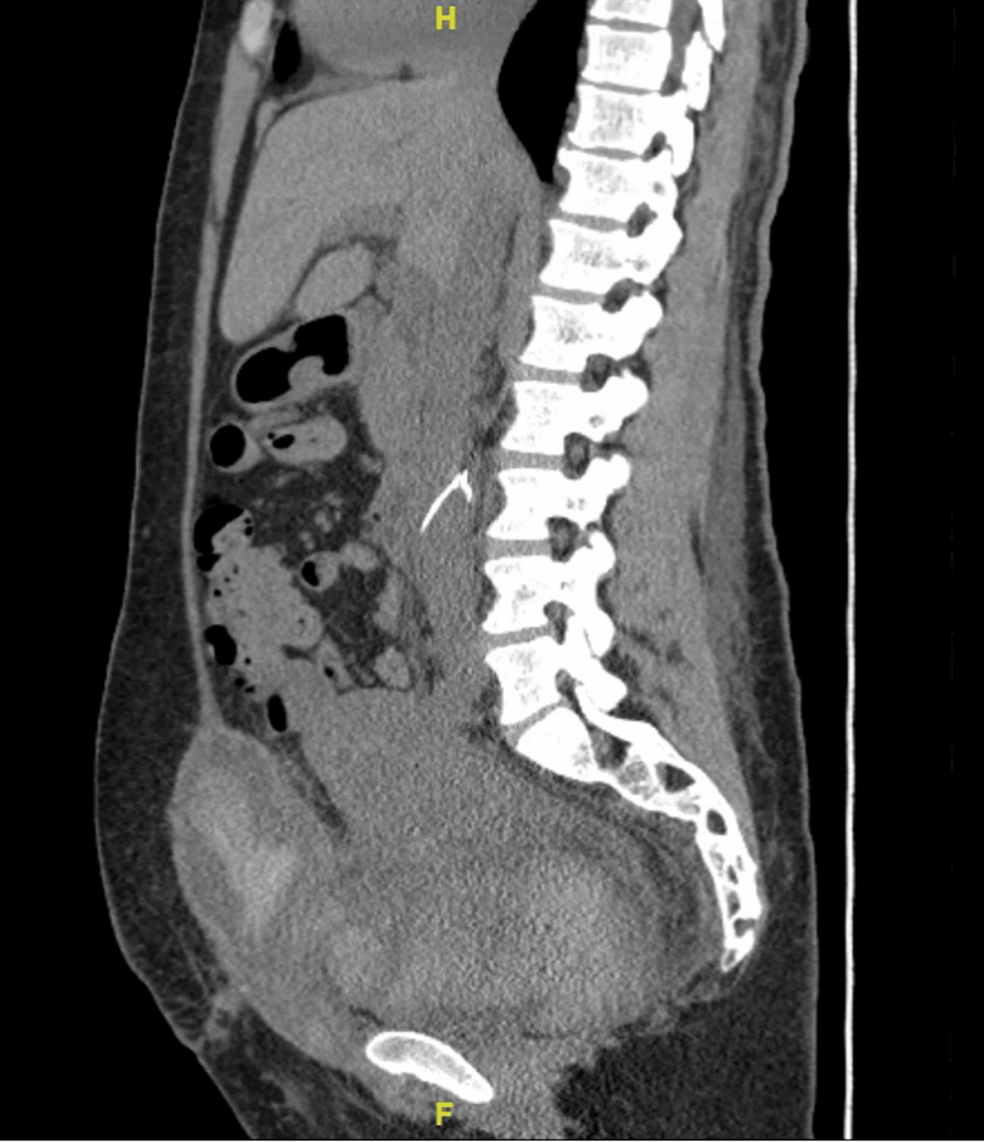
CT scan of the abdomen and pelvis showed bilateral nephrostomy tube placement with a resolution of hydronephrosis, renal cortical enhancement, and pelvic hematoma. Arrow shows pelvic hematoma
